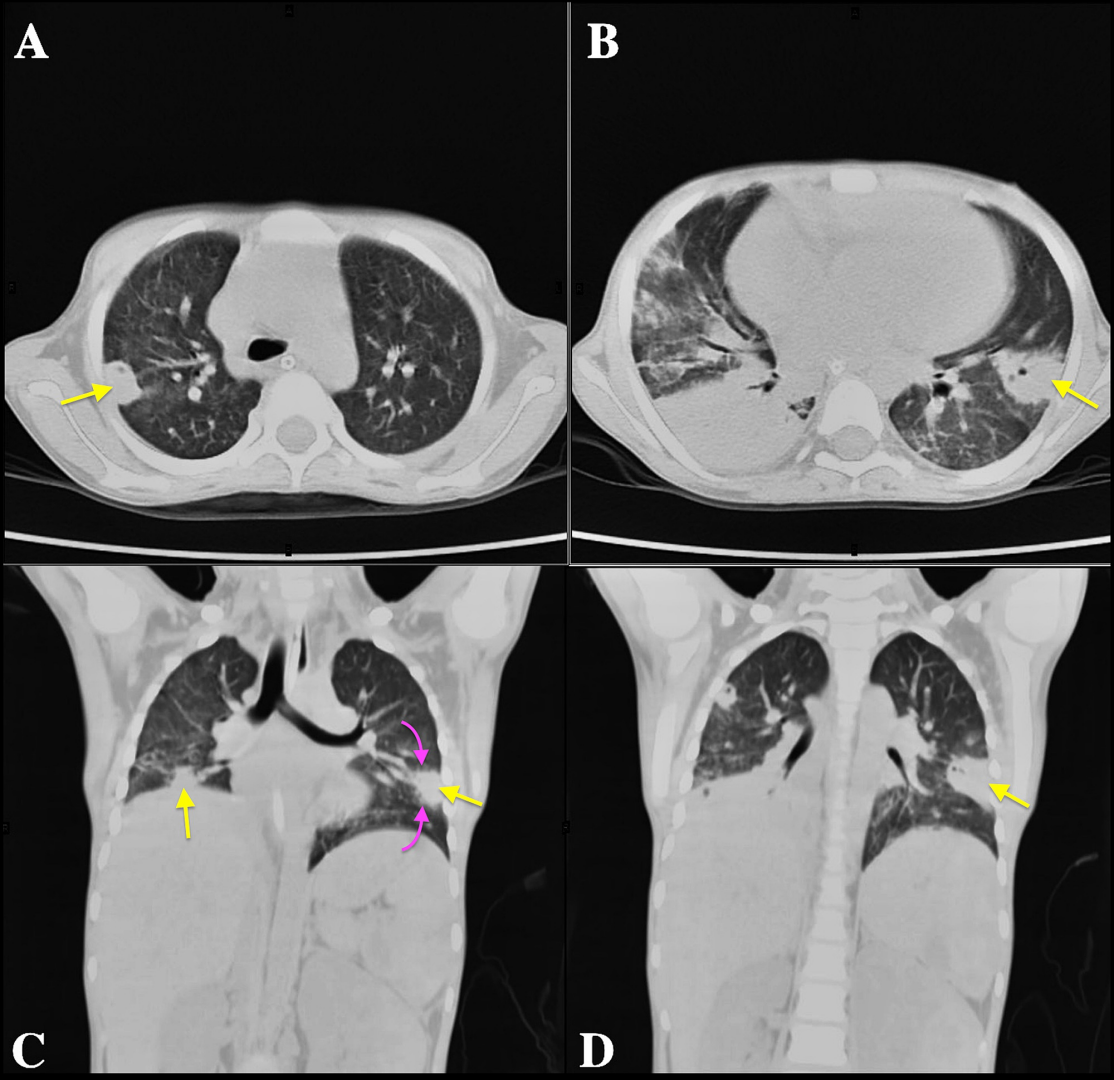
Computed Tomography (CT) of the chest without intravenous contrast demonstrate multiple peripheral nodular consolidations (yellow arrows) on transaxial (A and B) and coronal (C and D) lung windows suggestive of disseminated infection. Some of these nodules show internal cavitation indicative of necrosis. Few of them demonstrate surrounding ground-glass related to perilesional hemorrhage (purple arrows). In a patient undergoing chemotherapy, the favored diagnosis was an angioinvasive disseminated mycosis, and treatment with voriconazole was instituted.
